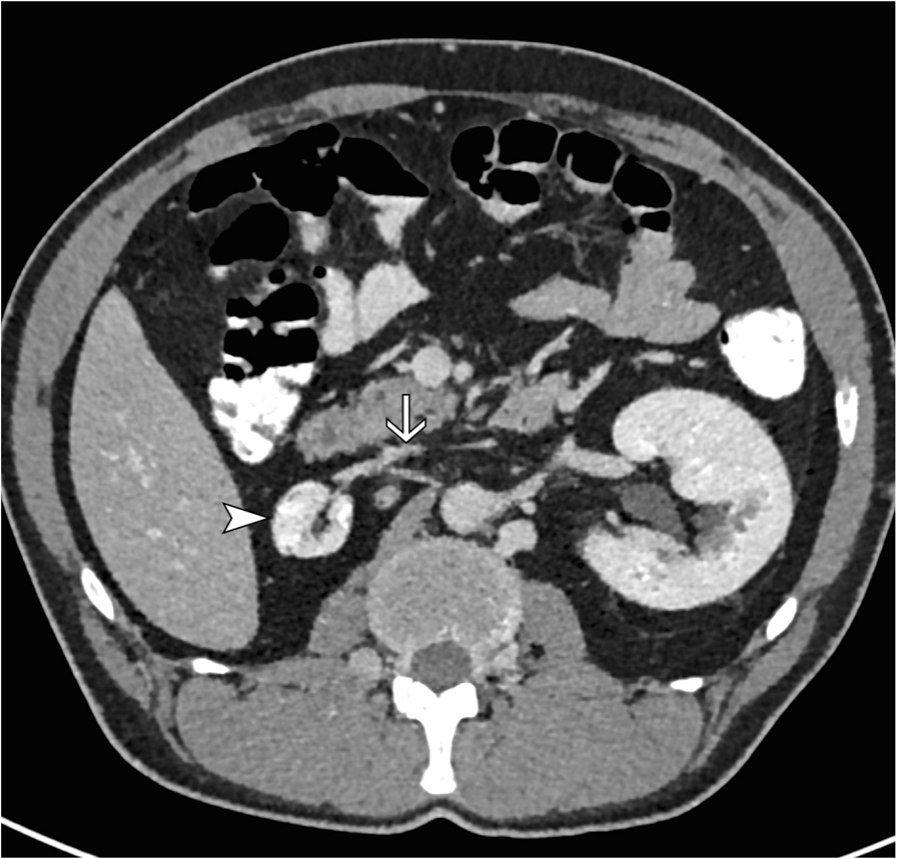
Abdominal CT Scan. CT shows shrunken right kidney (arrowhead) and IVC atresia (arrow)
